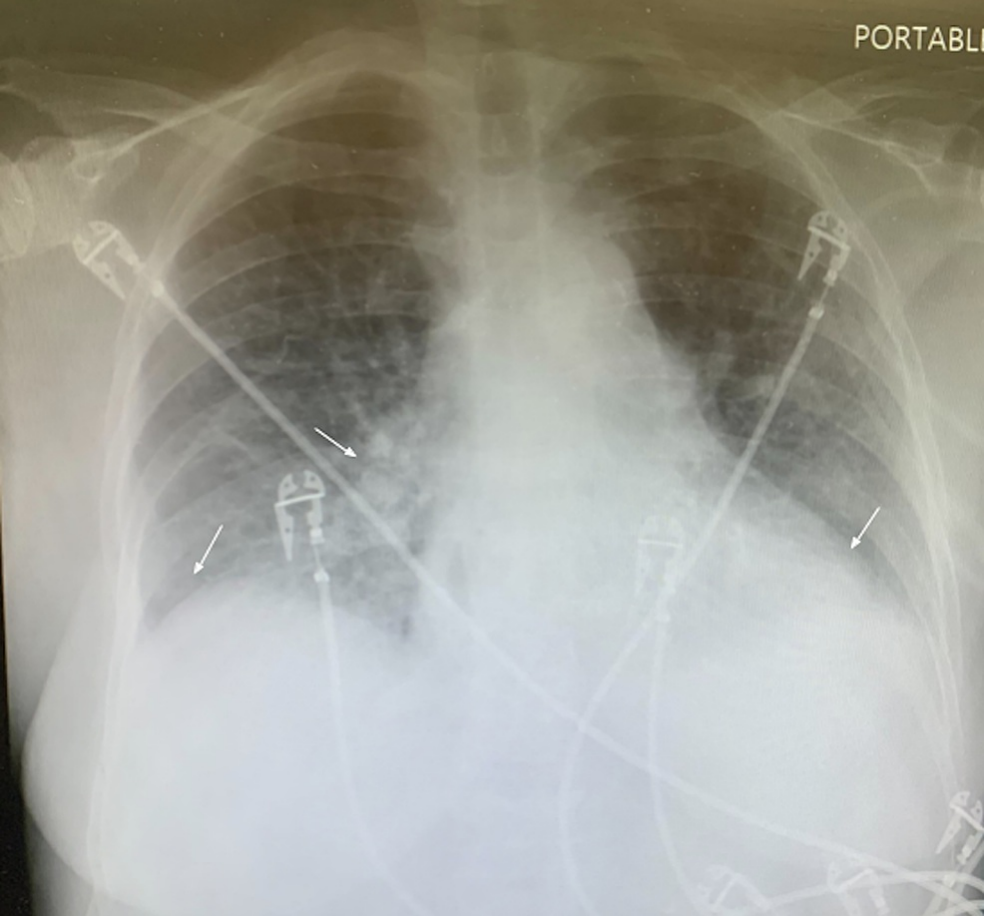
Portable chest x-ray demonstrating right lower lobe atelectasis, pulmonary vascular congestion, and cardiomegaly (arrows).
